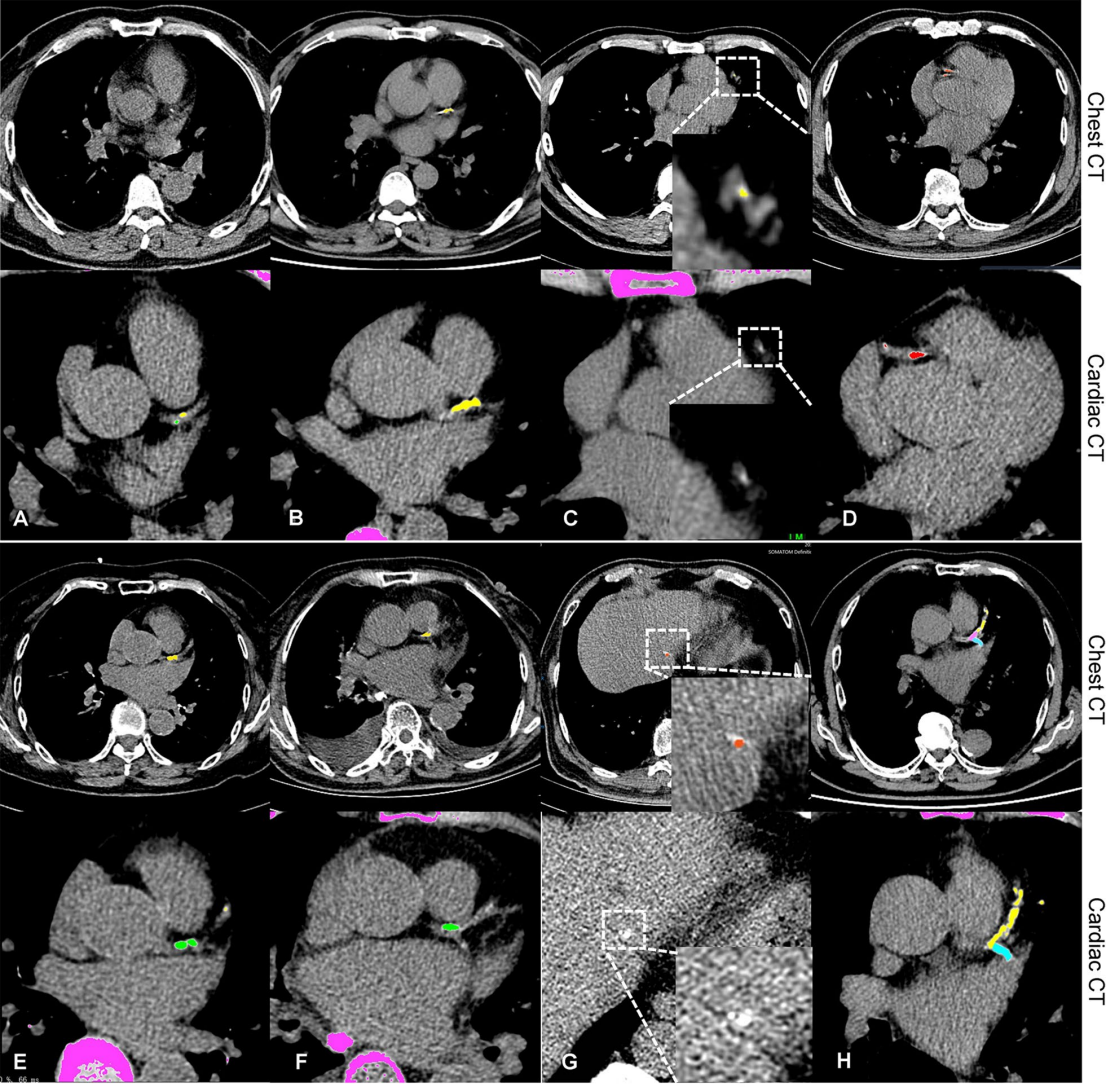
Examples show potential causes of discordances of Agatston scores between chest CT and cardiac CT. For each case(A-H), the upper row is AI-based scoring on chest CT, and the lower row is the manual measurement on cardiac CT. CAC lesions were annotated with different colours (i.e., green for LM, yellow for LAD, blue for CX, red for RCA, and pink for non-coronary calcifications). A. False negatives due to motion artifact, B. Underestimation due to motion artifact (Agatston score:57.7 versus 225.0 between chest CT and cardiac CT). C. False positive due to image noise on chest CT, it is not identified because of tiny size and lower density (< threshold of 130HU) on standard cardiac CT. D. Overestimation due to motion artifact (Agatston score:126.0 versus 88.0 between chest CT and cardiac CT). E. Segmentation error, CAC of LM (green on cardiac CT) is falsely identified as calcification of LAD (yellow on chest CT). F. Segmentation error combined with motion artifact, CAC of LM (green on cardiac CT) is wrongly identified as CAC of LAD (yellow on chest CT) and blurred due to motion artifact. G. Segmentation error, calcification of liver is misidentified as CAC of RCA. H. Segmentation error, part of CAC of LAD is misidentified as non-coronary calcification, resulting in underestimation (Agatston score:48.1 versus 713.2 between chest CT and cardiac CT)
